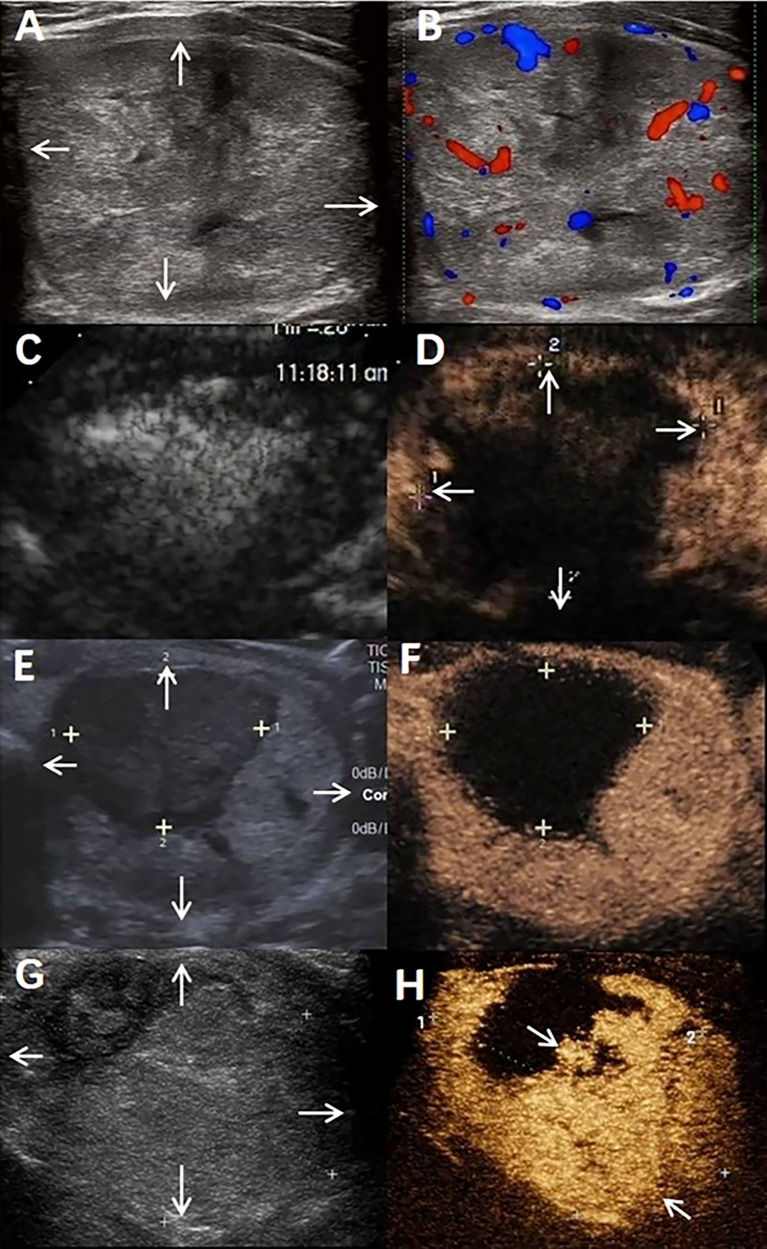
A benign thyroid nodule in a 44-year-old male treated with radiofrequency ablation (RFA). (A) The nodule was in the patient’s left lobe. Arrows indicate the boundaries of the nodule. The longest diameter was 6.2 cm, and the volume was 62.6 mL. (B) Colour ultrasound imaging of nodule. (C) Conventional ultrasound imaging of nodule Immediate after radiofrequency ablation. (D) Contrast-enhanced ultrasound imaging of nodule Immediate after radiofrequency ablation. Arrows indicate the boundaries of the treated area. The longest diameter of treated areas was 5.3cm and the volume was 36.5mL. IRAR is 58.3%. (E) Conventional ultrasound imaging of nodule at 6 months after radiofrequency ablation. Arrows indicate the boundaries of the nodule. The longest diameter was 5.0cm, the total volume of nodule was 37.2mL, VRR is 40.6%. (F) Contrast-enhanced ultrasound imaging of nodule 6 months after radiofrequency ablation. (G) Conventional ultrasound imaging of nodule at 12 months after radiofrequency ablation. Arrows indicate the boundaries of the nodule. The longest diameter was 5.2cm, the total volume of nodule was 35.1mL. VRR is 43.9%. (H) Contrast-enhanced ultrasound imaging of nodule 12 months after radiofrequency ablation. Arrows indicate the marginal regrowth of the nodule.
